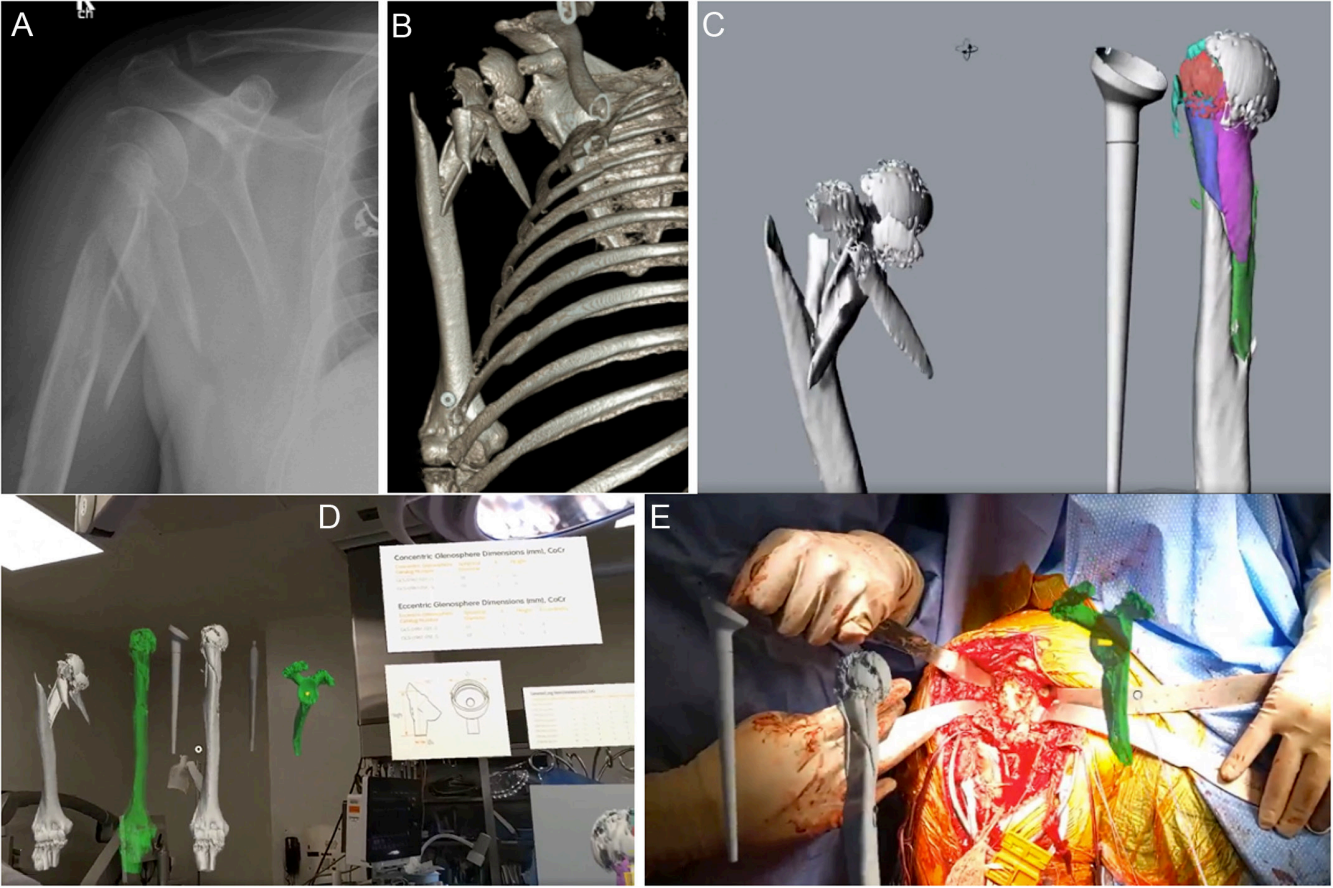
Proximal humerus fracture treated with reverse shoulder arthroplasty using mixed reality. (A) Initial injury anteroposterior (AP) radiograph, (B) 3D CT reconstruction of fracture,and (C) 3D planning to preoperatively plan fracture reduction and stem height. (D) Intraoperative holograms in the operating room, dragged and positioned in space. (E) Positioned holograms to assist in glenoid pin placement, stem placement, and fracture reduction.
